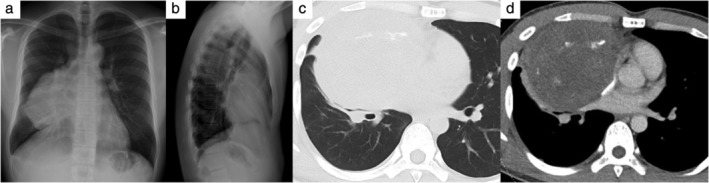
Chest radiography and computed tomography findings at the initial visit. Posteroanterior (a) and lateral (b) chest radiography findings showed an abnormal shadow in the right middle and lower lung field. Computed tomography findings revealed a well‐circumscribed tumor in the anterior mediastinum. (c) A pulmonary window image showed atelectasis in the right lung lobe. (d) Contrast‐enhanced computed tomography in a mediastinal window image revealed a heterogeneous tumor and some calcification.
